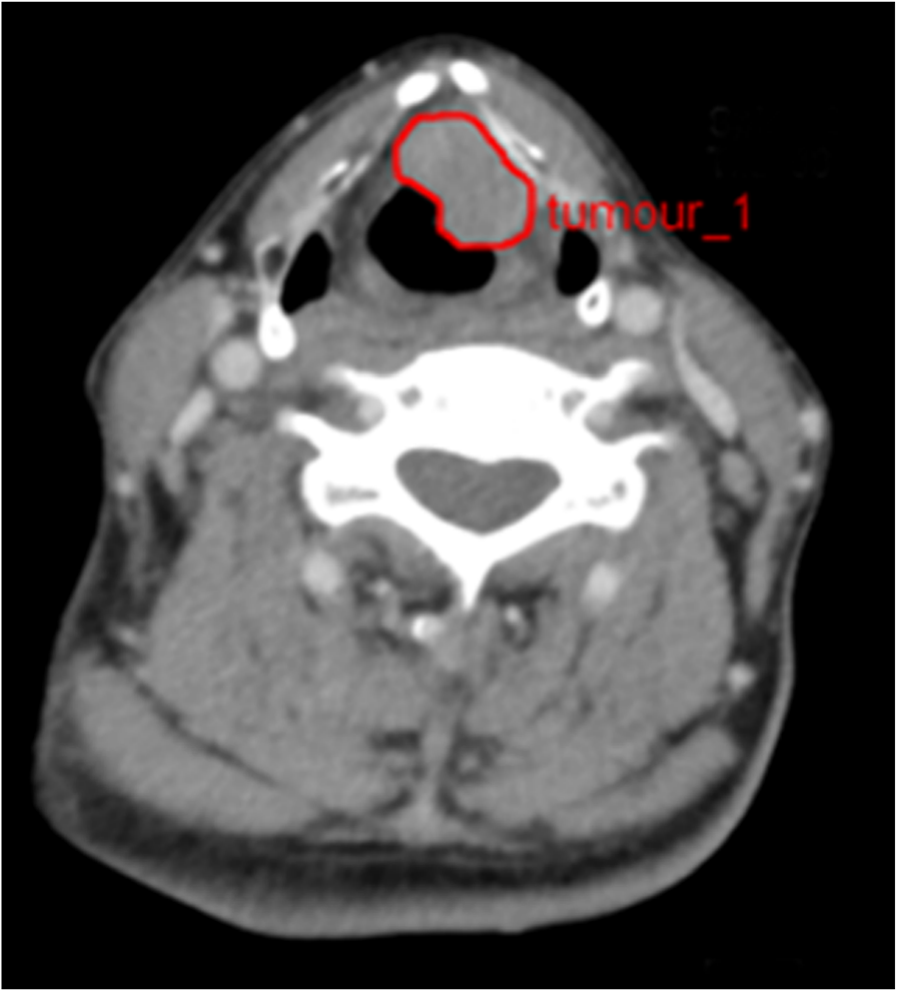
An example of manual segmentation in CE-CT image from a 65-year-old male patient with supraglottic laryngeal carcinoma. Red contour was drawn to contain the whole tumor region in one slice
